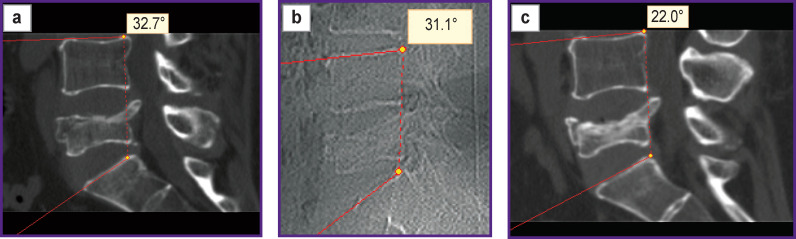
CT scan and X-ray examination of patient R., 45 years old: (a) 10 days after injury; (b) 6 months after the start of conservative treatment; (c) 12 months after the start of conservative treatment. Attention should be paid to the stabilization of the L_4_–S_1_ regional angle from the beginning of the follow-up to 6 months and its progressive decrease by 12 months
